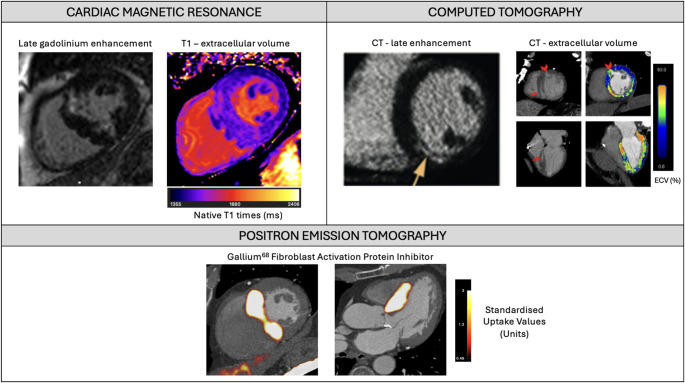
Existing and novel imaging approaches for measuring cardiac fibrosis. The current gold standard methods provide anatomical and physiological information (Upper Left). Cardiac magnetic resonance imaging can detect established replacement and diffuse interstitial fibrosis using surrogate parameters. Novel computed tomography markers have also recently developed which may also be able to detect established fibrosis but similarly to magnetic resonance, is only able to detect expansion of the extracellular space as a surrogate marker for fibrosis. By contrast, molecular imaging is able to detect myocardial fibrosis activity by detecting cellular markers specific for active fibrosis. One example is gallium-68 fibroblast activation protein inhibitor which binds to fibroblast activation protein, expressed on the cell surface of activated fibroblasts
